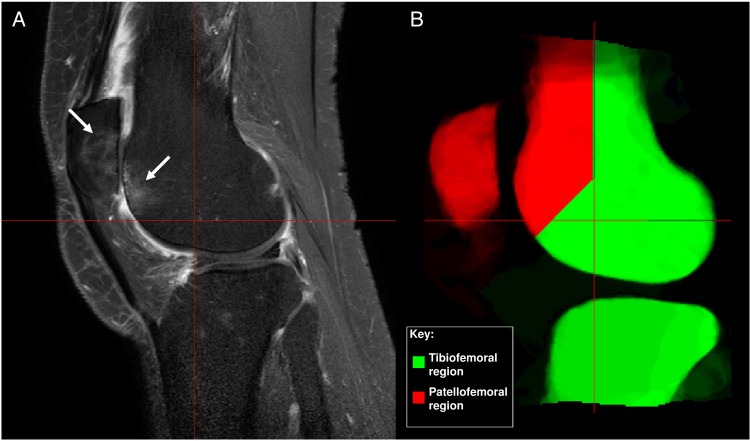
(A) Patellofemoral bone marrow lesions on MRI (arrows). (B) Regions defining patellofemoral and tibiofemoral bone marrow lesions.
